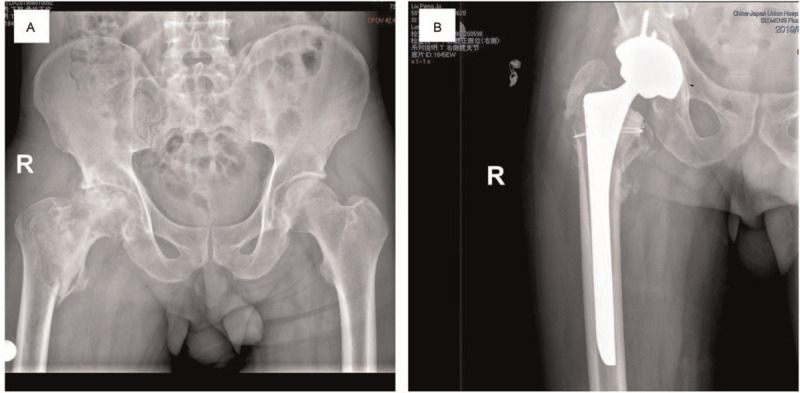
(A) Of an intertrochanteric fracture in a 58-year-old man with preexisting ipsilateral femoral head necrosis. (B) Postoperative radiograph shows he received a THA and immobilized the greater trochanter with metal cable. THA = total hip arthroplasty.
